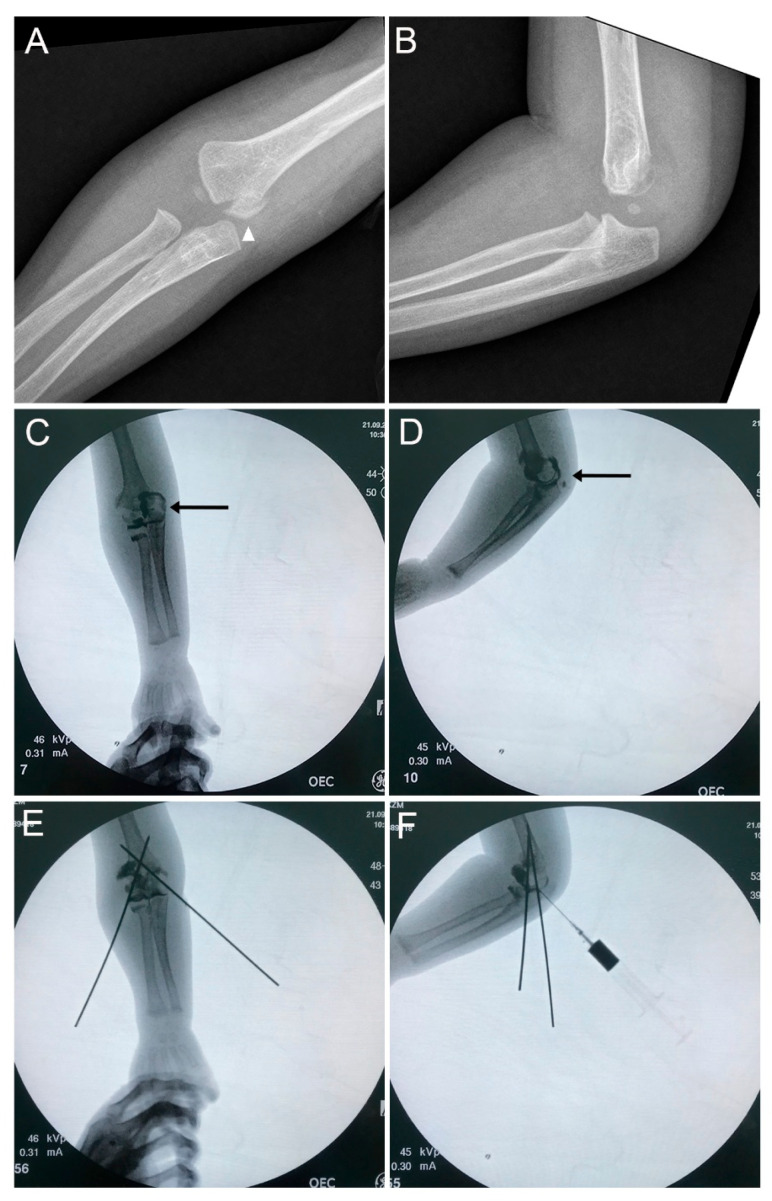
Transphyseal distal humerus fracture (TFDH) misdiagnosed as a medial condyle fracture. A 12-month-old boy (Case 12) was initially misdiagnosed with a medial condyle fracture. Soft-tissue swelling of the both medial and lateral elbow is present. (A,B): Initial anteroposterior and lateral radiographs. White arrowhead denotes the metaphyseal fragment (Thurston–Holland sign). (C,D): Arthrograms show posteromedial displacement of the distal humeral epiphysis with intact articular cartilage (black arrows). (E,F): Closed reduction and percutaneous pin fixation performed under arthrography guidance.
